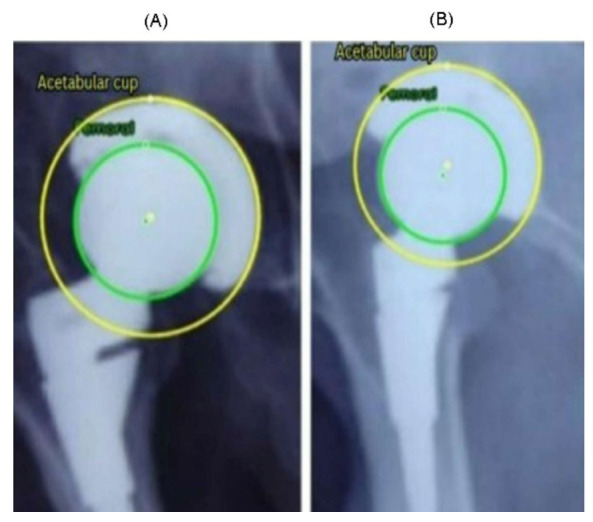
Example 2 of PRE–POST radiographs with detected femoral head and AC circles. (A) PRE radiograph demonstrating a centered femoral head within the acetabular cup. (B) POST radiograph showing superior displacement of the femoral head consistent with wear progression.
